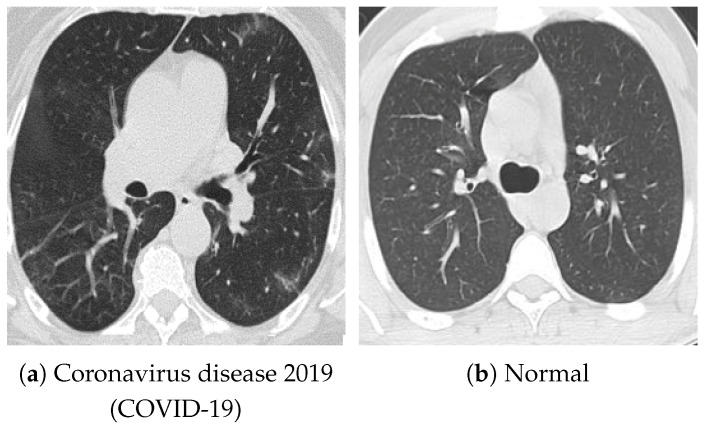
Examples of chest CT images on the SARS-CoV-2 CT-Scan dataset: (a) COVID-19 and (b) Normal.
